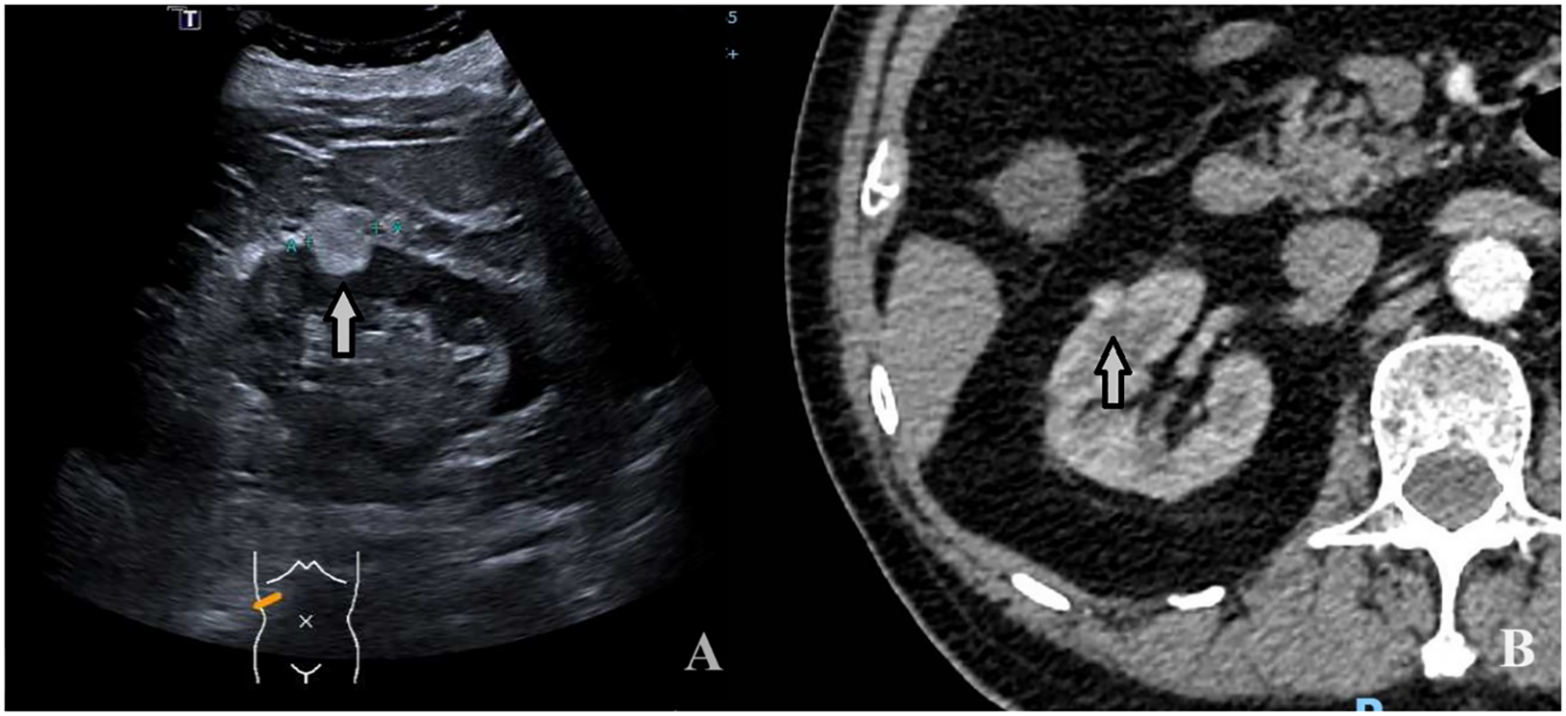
A 74-year-old man with a 1.9 × 1.4cm RAML. (A) Ultrasound demonstrated a mildly hyperechoic mass located in the middle pole of the right kidney (arrow). (B) CECT: the mass showed inhomogeneous hyperenhancement (arrow).
